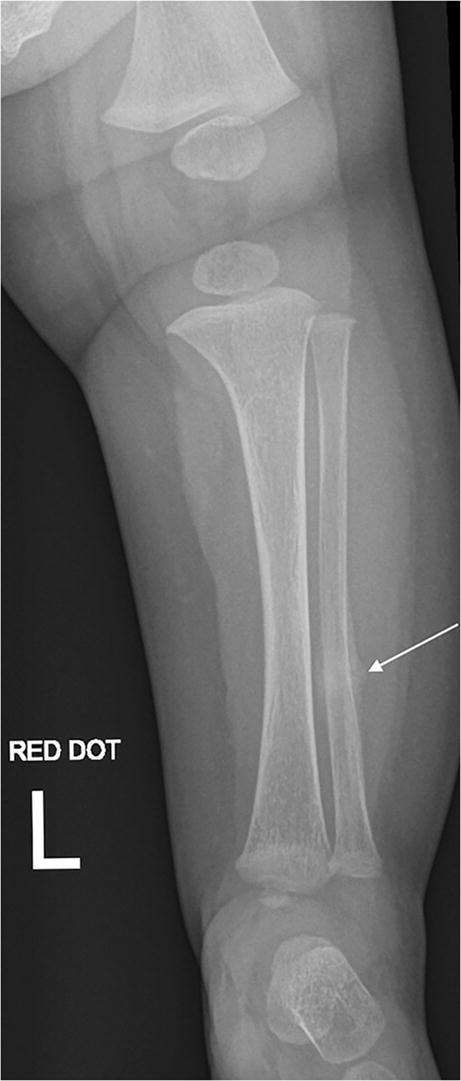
An anteroposterior radiograph of the left tibia and fibula was performed 2 days after Fig. 1, repeated due to persistent symptoms and initial “normal” radiographs. There is increased periosteal reaction (*arrow*) compared to the previous radiographs, consistent with progressive healing of an undisplaced fracture. There is normal density and modelling of the imaged bones
